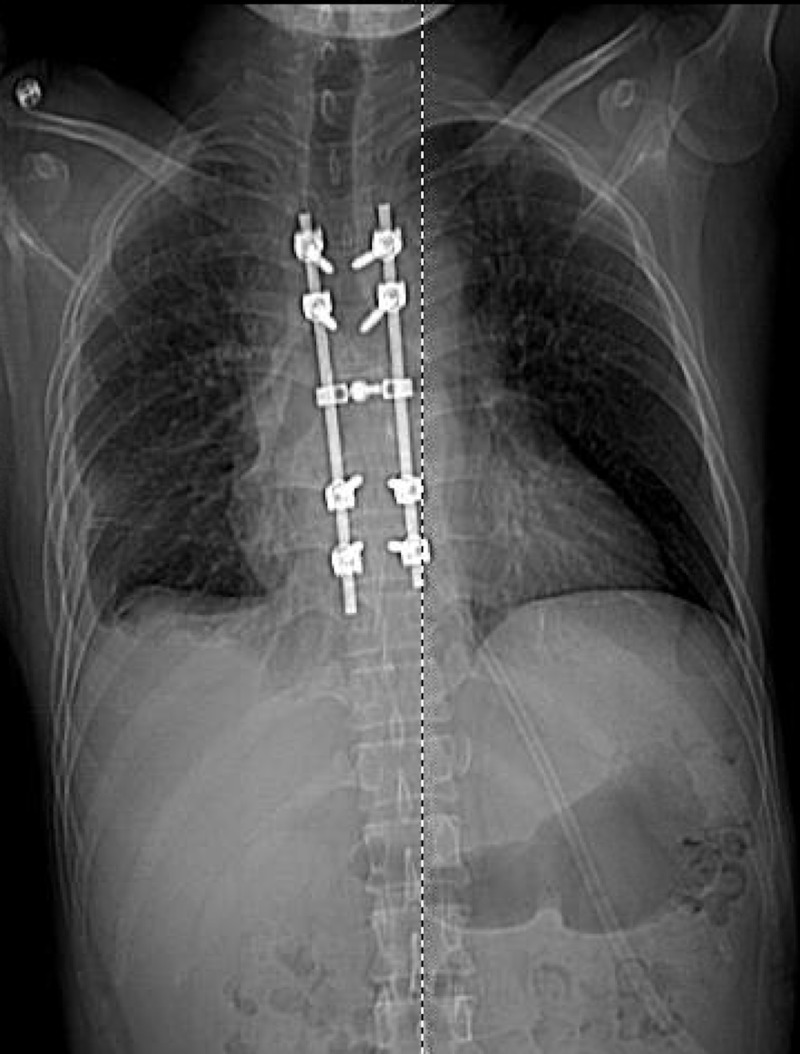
Postoperative radiograph Postoperative radiograph, anteroposterior view, shows thoracic hardware from T4-9 levels.
